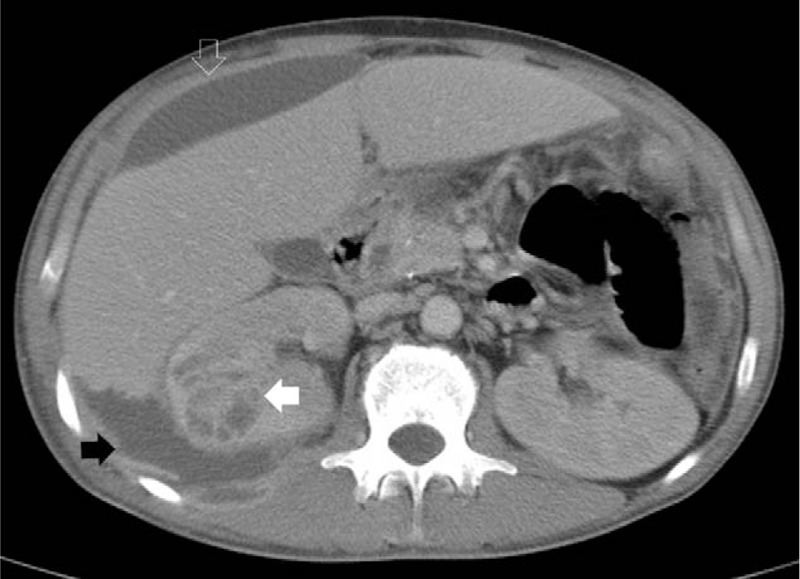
Abdominal computed tomography scan demonstrating multiple cystic renal abscesses with wall thickening (white arrow), irregular renal margin, fluid accumulation at the right subphrenic (white hollow arrow), and retroperitoneal (black arrow) spaces.
